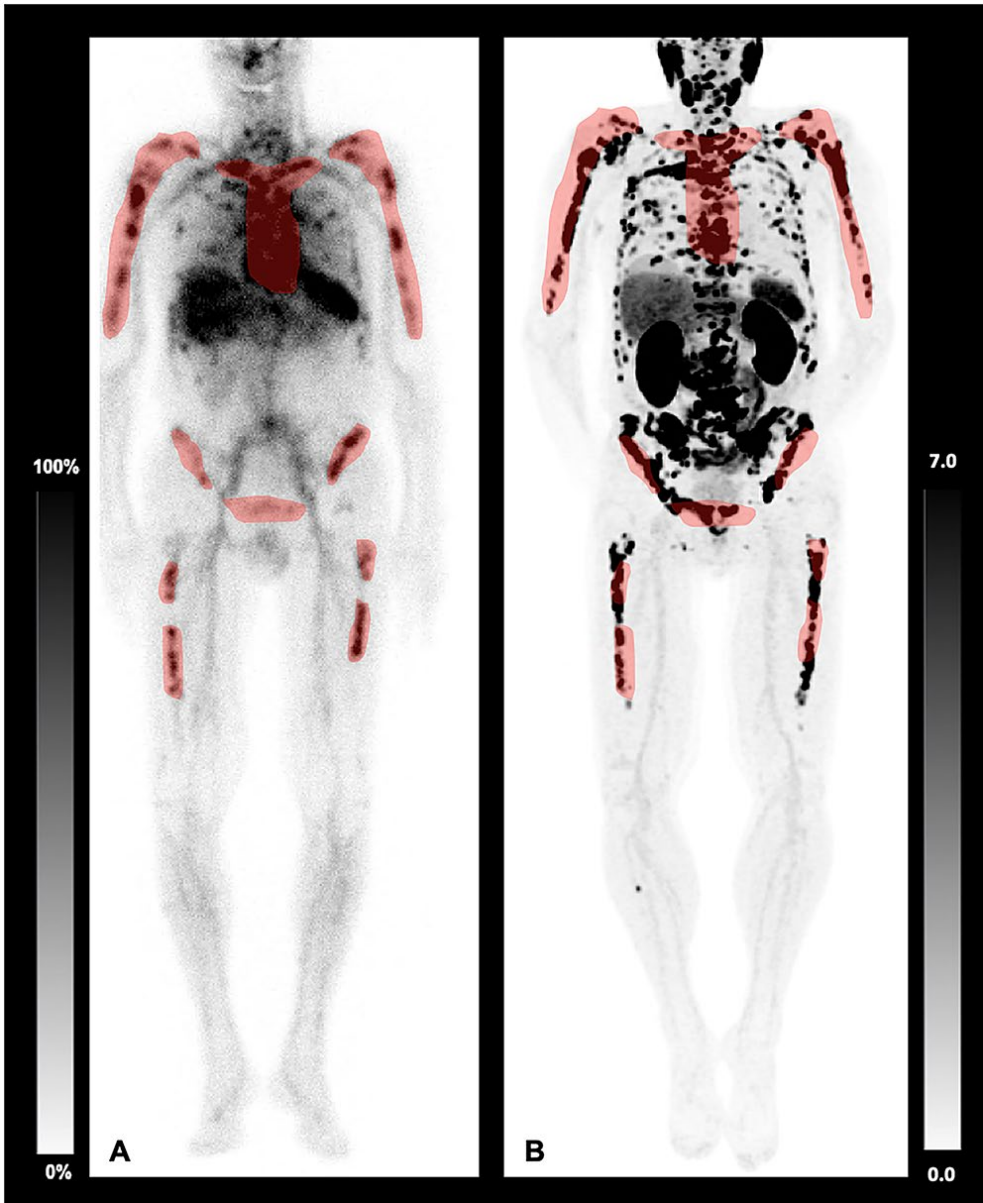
81-year-old patient prior to PSMA-RLT undergoing A) [^99m^Tc]Tc-antigranulocyte scintigraphy and B) [^18^F]F-PSMA-PET/CT presenting with congruence of viable bone marrow and osseous metastases, as demonstrated in red. Moderate bone marrow expansion is observed in the proximal extremities. A comparison of the results of the PSMA PET/CT scan with the other imaging techniques reveals extensive co-localization of the areas with hematopoietic bone marrow and metastatic infiltration. Nevertheless, more extensive areas of hematopoietic bone marrow that are not subject to metastatic infiltration cannot be identified. The pronounced augmentation of the spleen is in accordance with the phenomenon of extramedullary hematopoiesis
